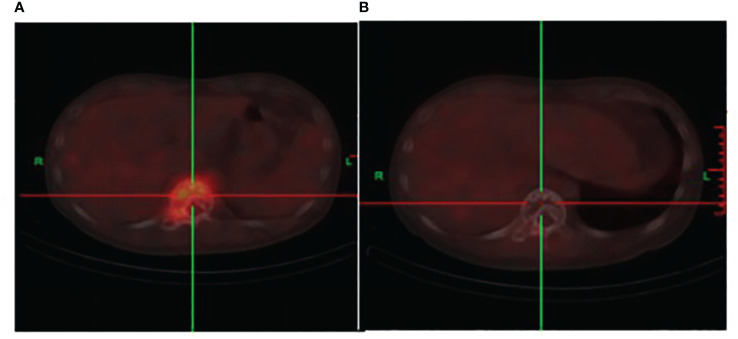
(A) The initial 18F-FDG co-registered imaging depicts bone destruction in the 11th thoracic vertebra. An occurrence of soft tissue shadows and an increase in metabolism are also observed. (B) After two cycles of DKRd treatment, the 18F-FDG displays bone destruction shadows in the 11th thoracic vertebra, with no significant signs of hypermetabolism, the metabolic shadow is disappearance.
